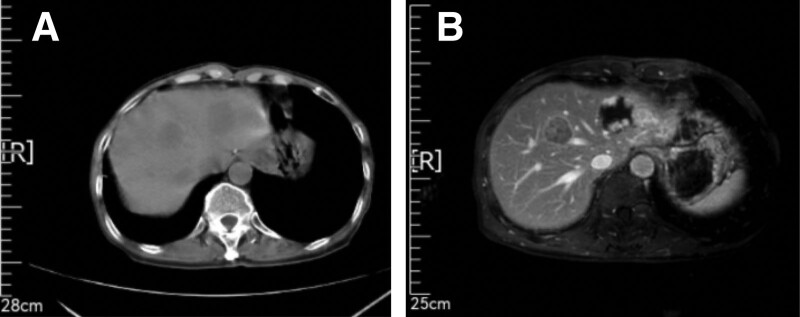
CT examination on March 16, 2021 revealed intrahepatic nodules, while MRI examination on March 16, 2021 revealed S4 segment nodules in the liver. Considering the possibility of metastasis based on medical history. Besides, multiple hemangiomas were found in the left lobe of the liver. CT = computer tomography, MRI = magnetic resonance imaging.
